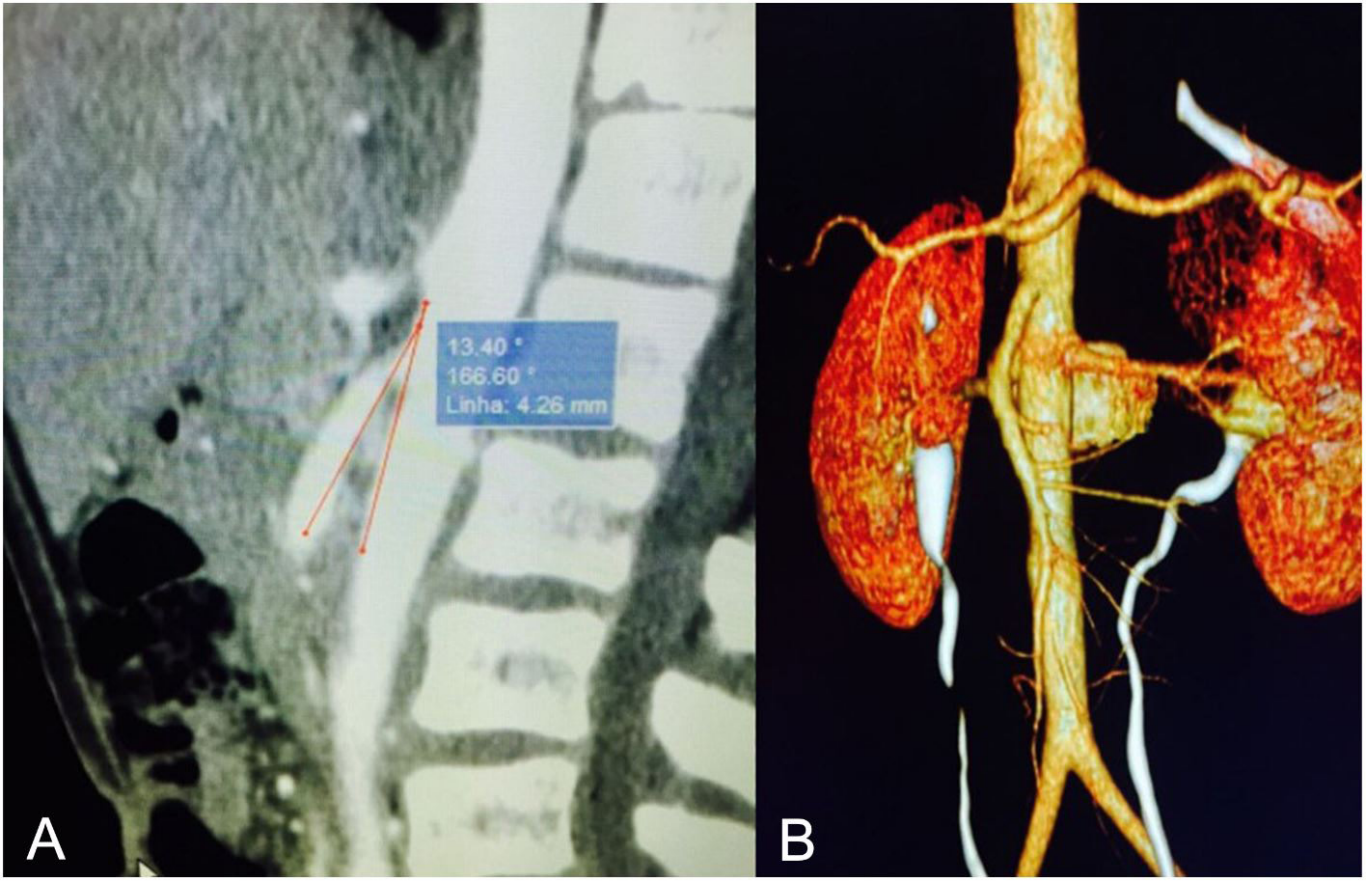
The initial computed tomography (A) and three-dimensional reconstruction (B)
